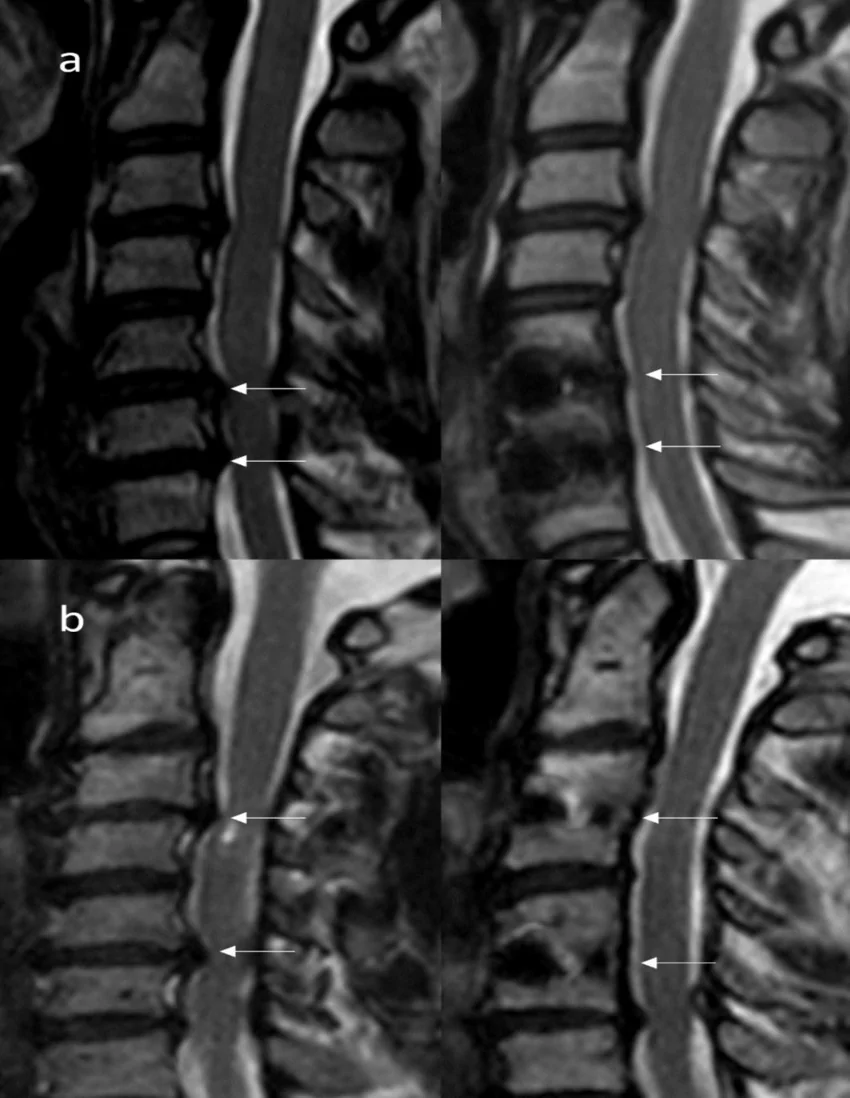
Representative preoperative and postoperative MRI findings. Sagittal T2-weighted MR images obtained before (left) and after (right) surgery in representative patients with (a) Modic changes and (b) without Modic changes. Arrows indicate the surgical level, demonstrating satisfactory decompression of the spinal cord following ACDF.
